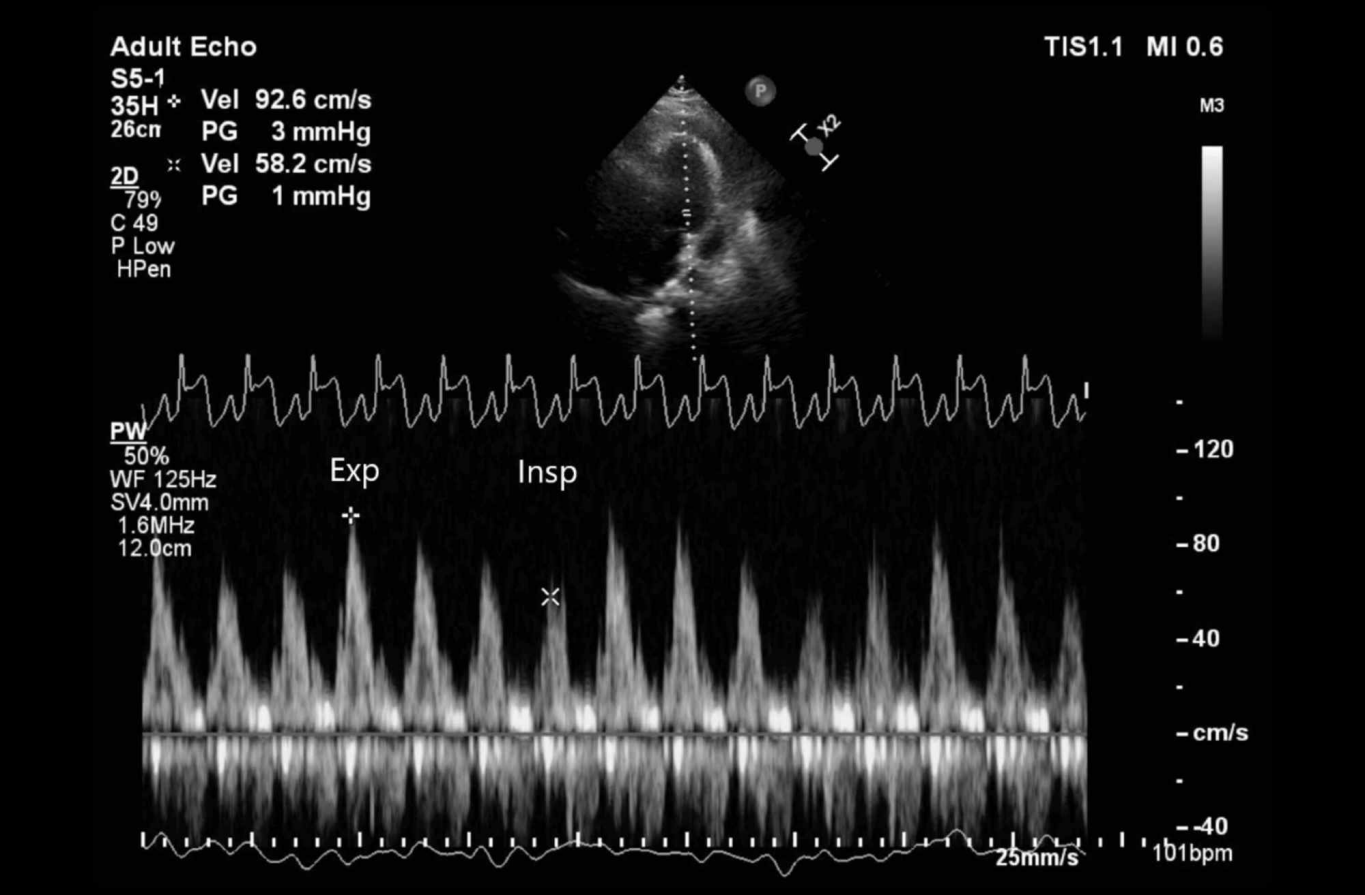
Pulse-wave Doppler of left ventricular inflow on transthoracic echocardiogram (TTE) Pulse-wave Doppler of left ventricular inflow at mitral valve level on TTE demonstrates prominent inspiratory reduction in flow (Insp) compared to expiration (Exp), consistent with tamponade physiology.
